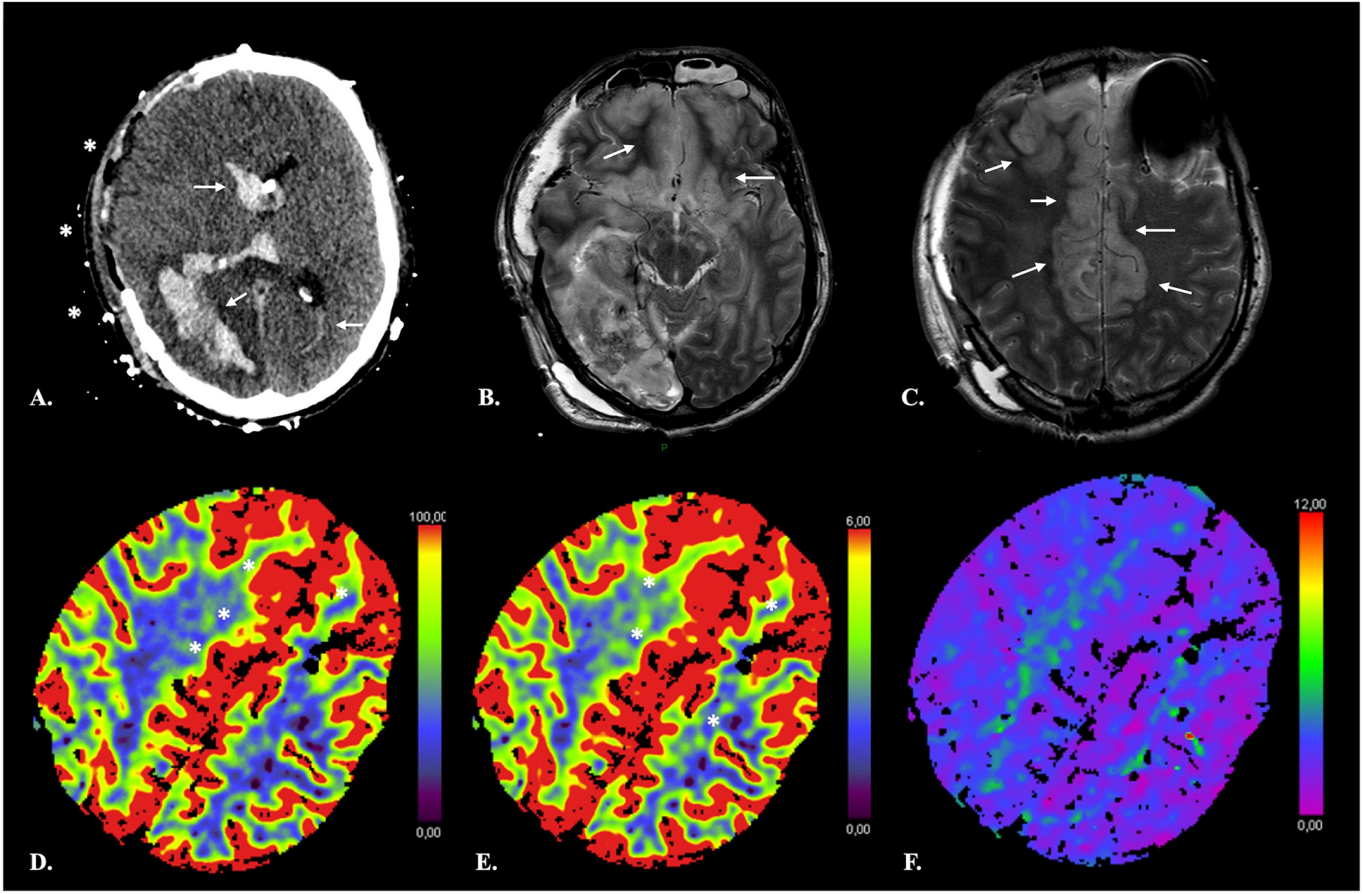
False-negative CTP results in a 41-year-old male with extensive ischemia of the anterior cerebral cortex due to subfalcine herniation following rupture of a right parieto-occipital AVM. NECT of the brain (A) shows a subacute right parieto-occipital hematoma with associated intraventricular hemorrhage (*arrows*). Midline shift has regressed following right hemispheric craniectomy (*). Axial T2-weighted TSE images (B,C) reveal swelling and increased signal intensity of the cortex in the ACA territory bilaterally consistent with ischemia. On CTP, the infarcted cortex displays increased CBF (D) and CBV (E) with no obvious TMax (F) abnormality resulting in negative computation of both NVT and TAR by the software.
